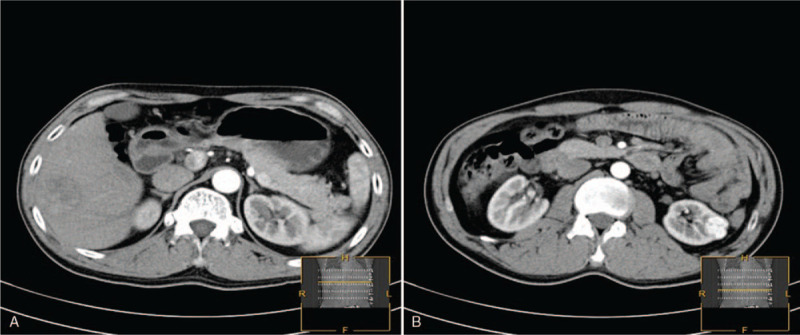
CT prior to nephron-sparing surgery of the left kidney and radical partial excision of the right liver lobe. (A) The enhanced computed tomography (CT) scan revealed a mildly enhanced tumor measuring approximately 3 × 4 × 5 cm^3^ in the right lobe of the liver. (B) Another significantly enhanced mass measuring approximately 2 × 3 × 3 cm^3^ was observed in the inferior pole of the left kidney without local invasion or lymph node metastases, and no mass lesions were found on the bilateral adrenal glands.
